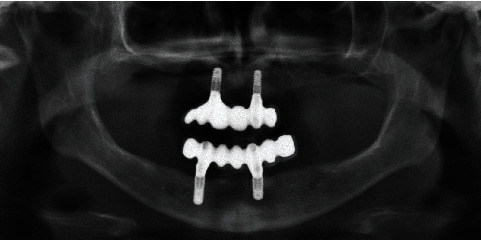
Panoramic radiograph (OPG) taken one year after the placement of the definitive restorations. The framework of the extremely shortened dentition (ESDA) is clearly visible, and the peri-implant bone conditions show no signs of inflammation.
